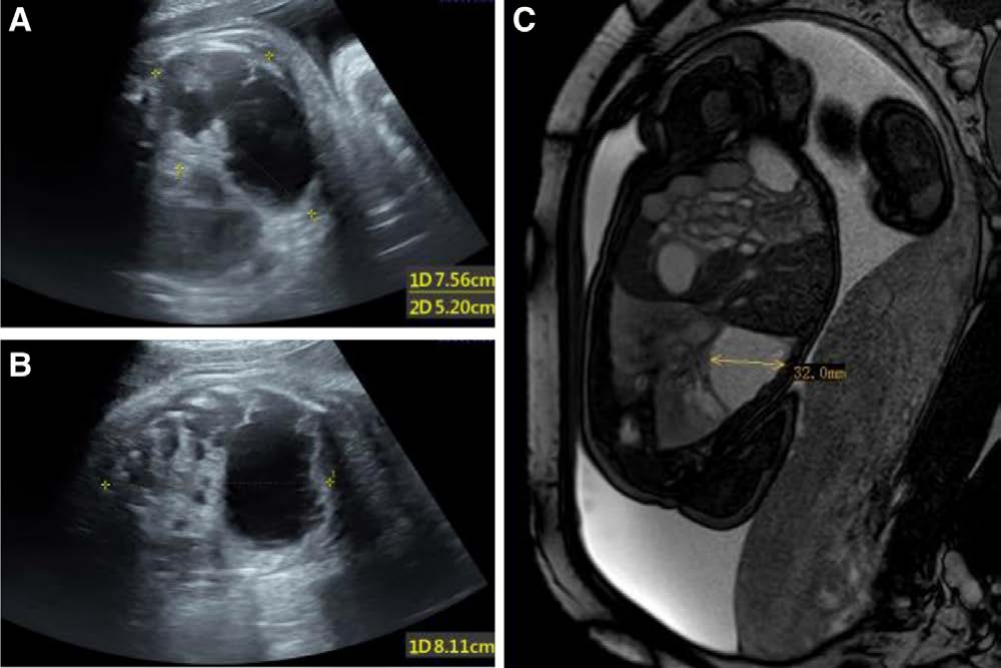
(A) and (B) Antenatal ultrasound at 35 wk gestational. (C) MRI of the uterus and the fetus at 34 wk gestational. MRI = magnetic resonance imaging.
